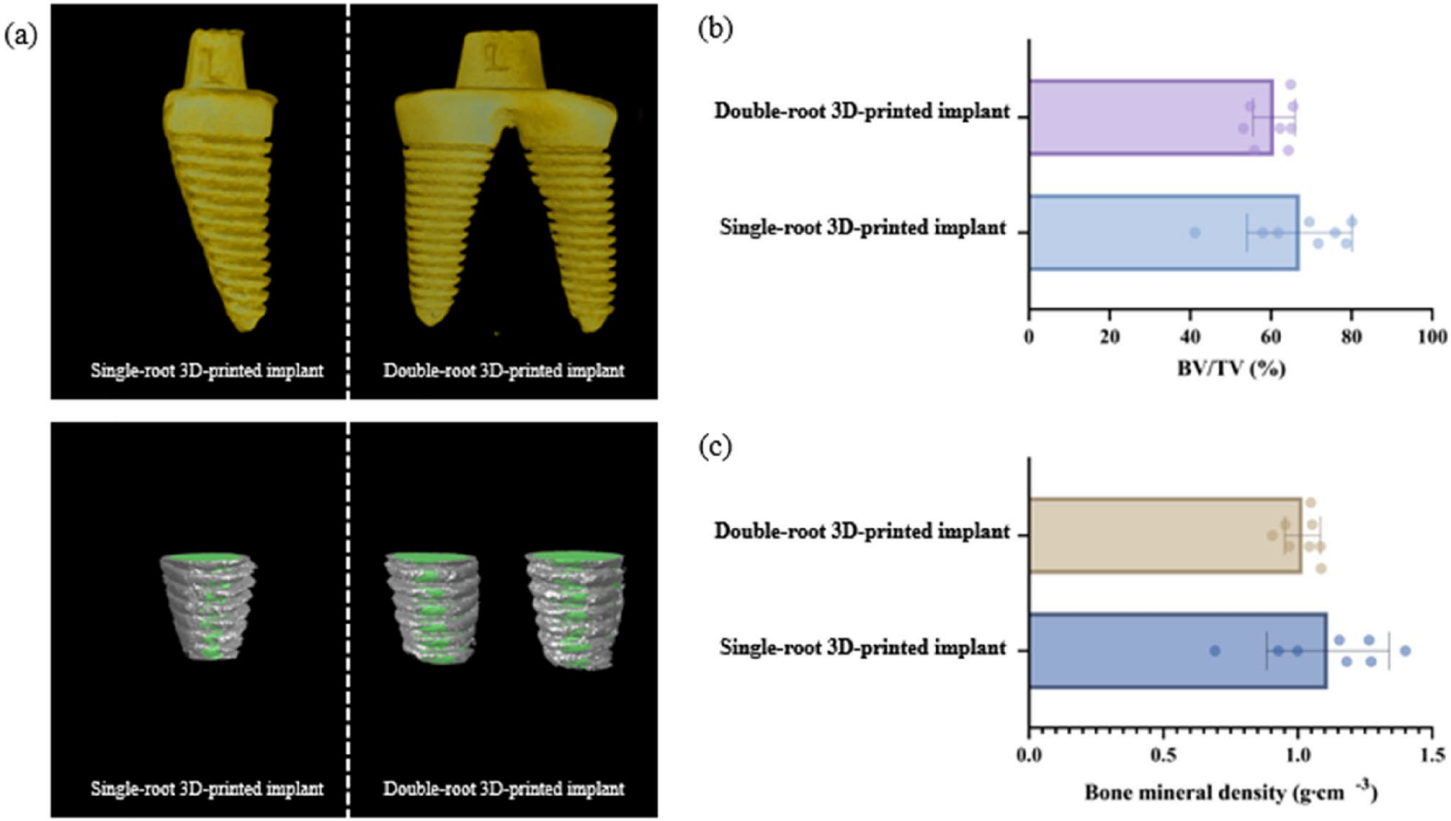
Representative micro-computed tomography (a) and analysis (b, c). (a) Gray and green areas indicate the VOI and 3D-printed implants, respectively. BMD and BV/TV were measured in the gray area. (b) The BV/TV of single-root and multi-root 3D printed implant were 67.11 ± 13.05% and 60.76 ± 5.43%, respectively, showing no statistically significant difference. (c) The bone mineral density of single-root and multi-root 3D printed implant were 1.11 ± 0.23 g mm^−3^ and 1.02 ± 0.08 g mm^−3^, respectively, showing no statistically significant difference. CT: computed tomography, VOI: volume of interest, 3D: 3-dimensional, BMD: bone mineral density, BV/TV: bone volume/tissue volume.
